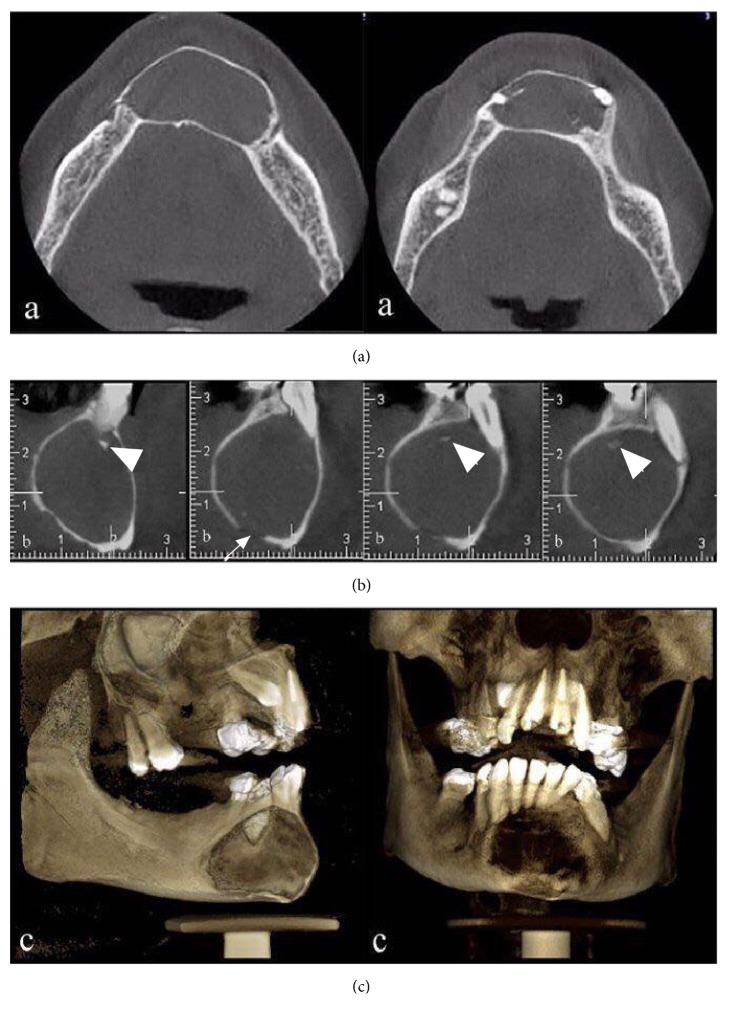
(a) Axial sections show that mental foramen is not involved but has close contact with border of the lesion at the left side. (b) Cross-sectional CBCT images reveal radiopaque spots inside the lesion indicated by white arrows in the image. (c) Three-dimensional volumetric surface rendering.
